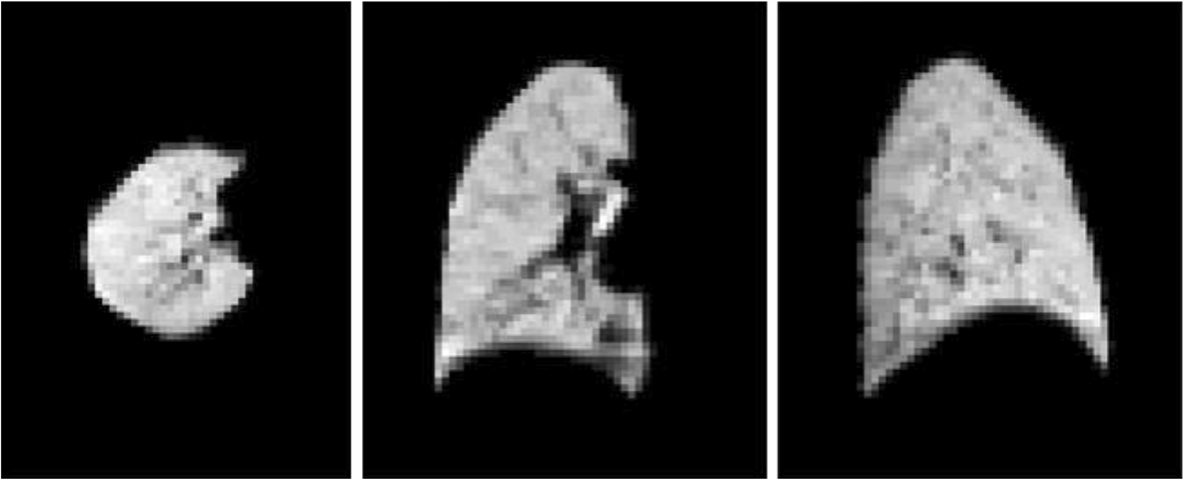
Example 2D transverse, coronal and sagittal sections from a 3D volume image obtained from a CT scan.
